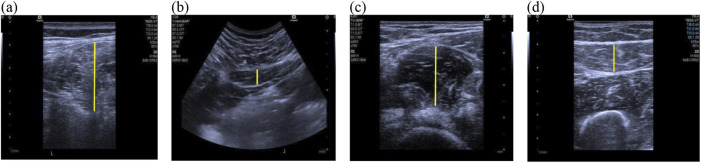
Ultrasonographic Images of muscles at rest. (A) Erector spinalis; (B) Quadratus lumbalis; (C) Iliac muscle; (D) Rectus femoris; the yellow lines represent the measurement range of muscles thickness.
